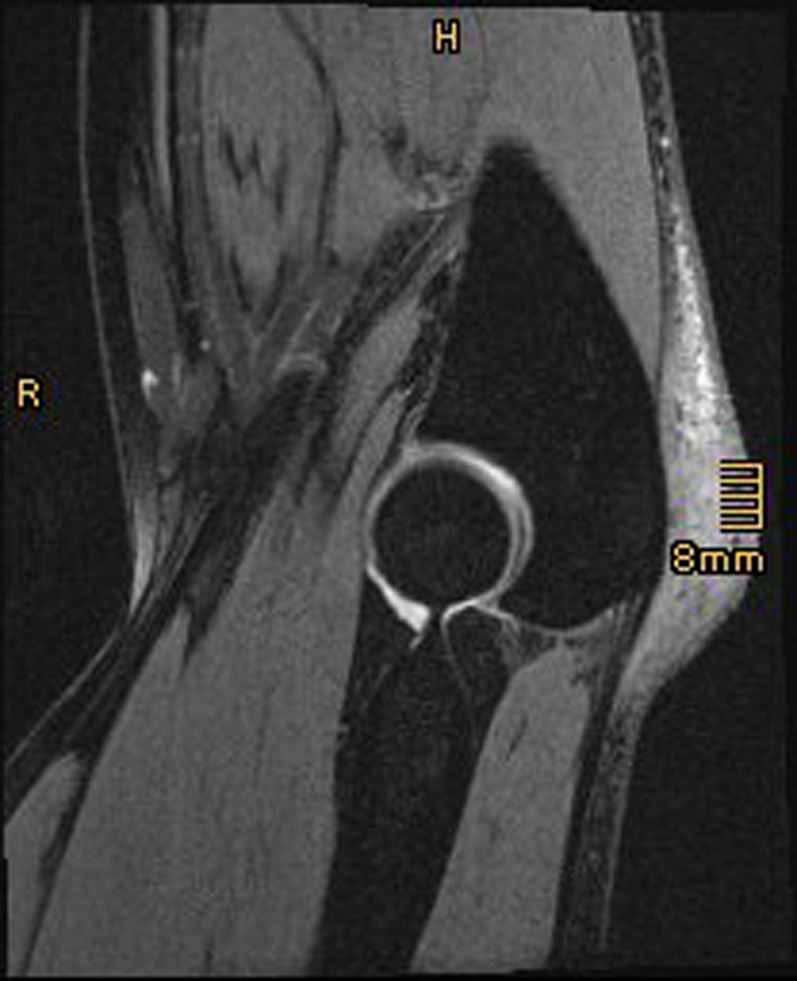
Sagittal T2-weighted MRI of a 35-year-old male patient’s right elbow showing olecranon bursitis with inhomogeneous signal enhancement of the thickened olecranon bursa.
